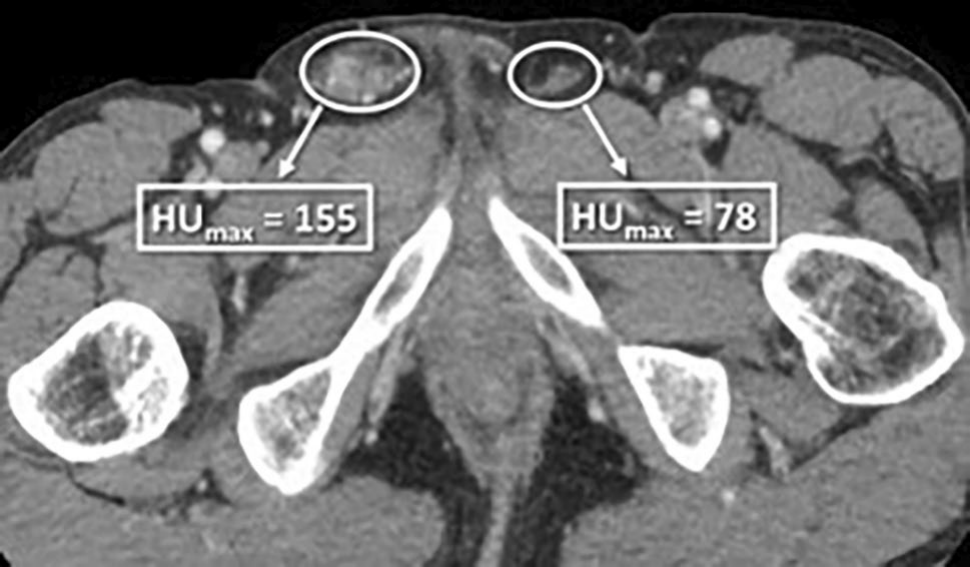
58-year-old with postoperative pelvic and scrotal swelling and pain with one of two contiguous axial CT images best depicting spermatic cord shown with ROI’s encompassing the cords. Differential enhancement was 50% higher on the right in this patient with right-sided epididymitis
